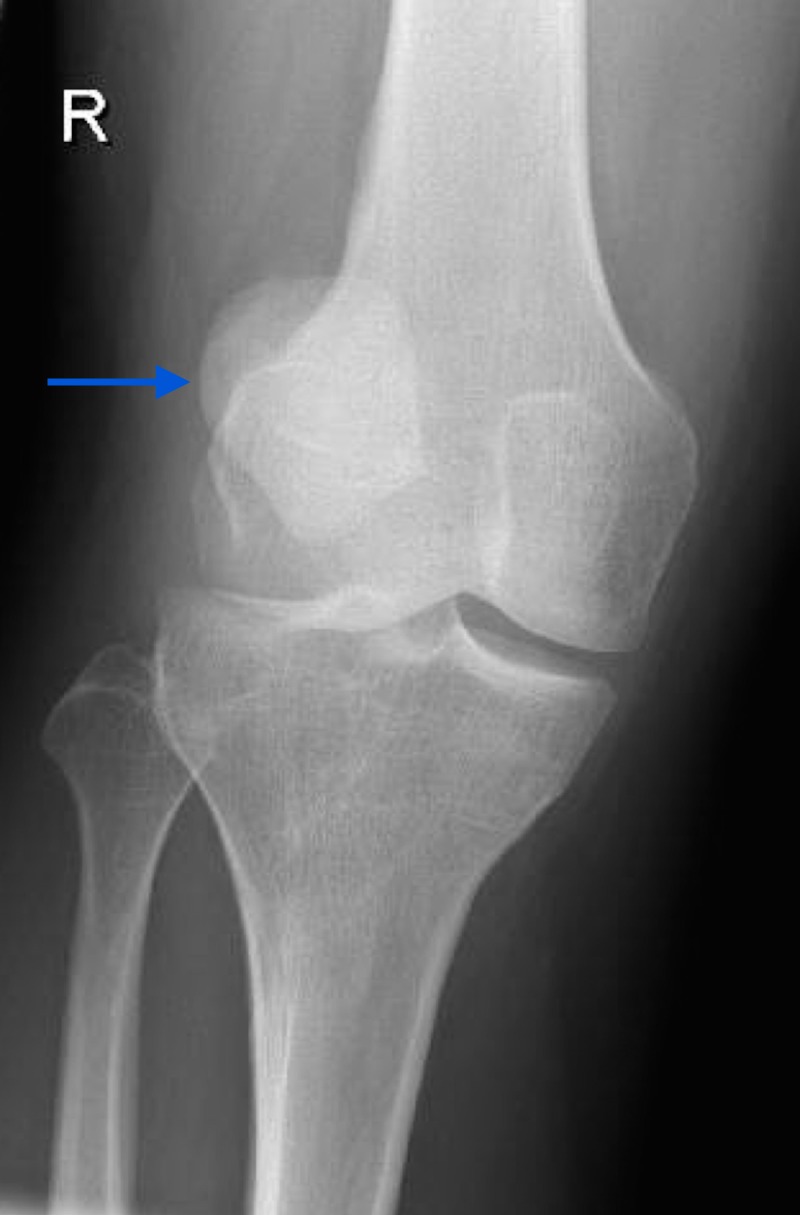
Plain anteroposterior radiograph of right knee showing lateral patellar displacement (blue arrow).
